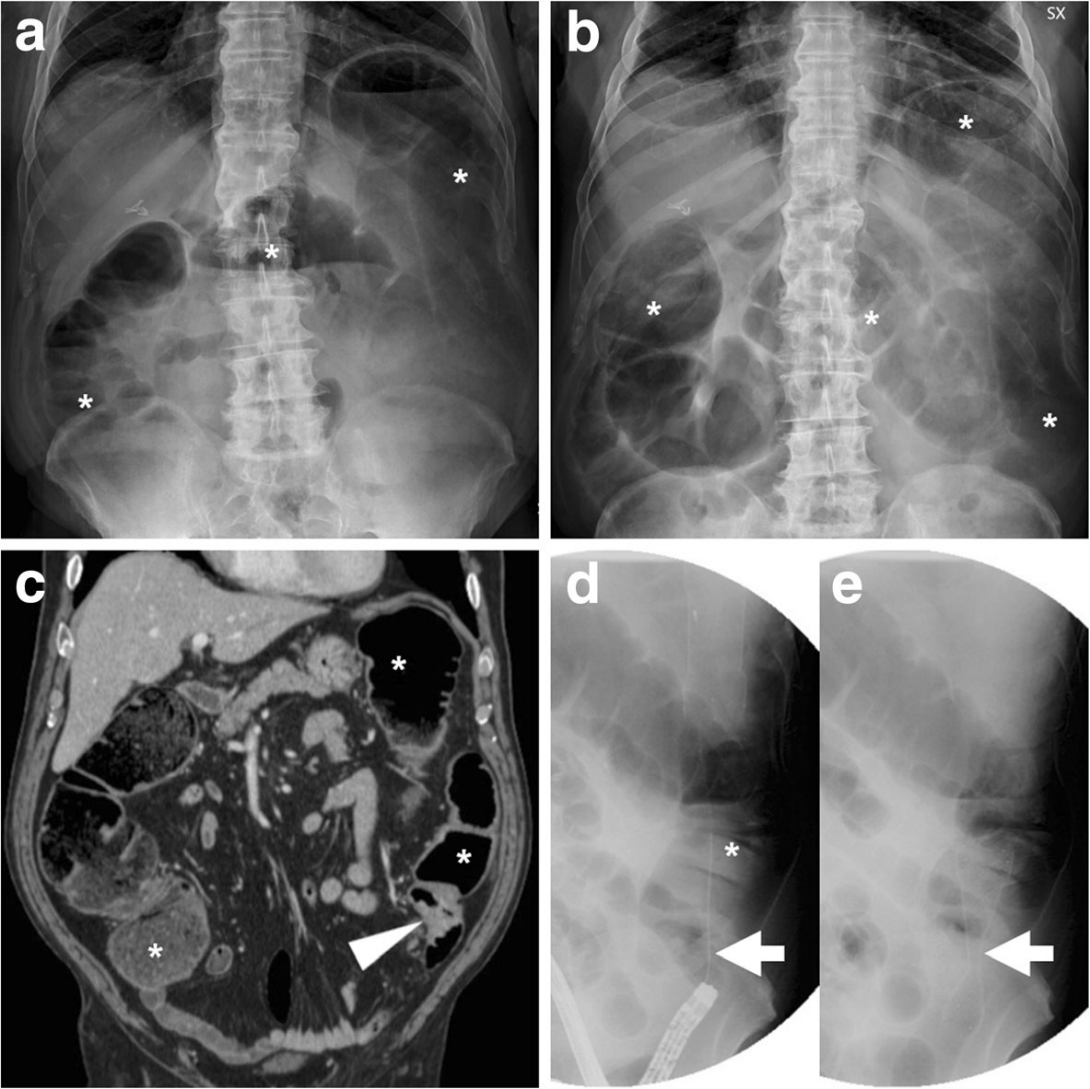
“Bridge-to-surgery” stenting of obstructing CRC. Upright (a) and supine (b) plain abdominal radiographs show marked dilatation of the large bowel (*) with fluid-faecaloid material consistent with mechanical LBO (note air-fluid levels in a). Coronal CT image (c) confirm colonic dilatation (*) above a 4-cm-long, solid mural thickening at the distal descending colon (arrowhead). After biopsy, fluoroscopic images documented the endoscopic positioning of a SEMS (thick arrow in e) along the guidewire (thick arrow in d) through the tumour. The patient then underwent left hemicolectomy with primary anastomosis to remove pT3N1G2 carcinoma
